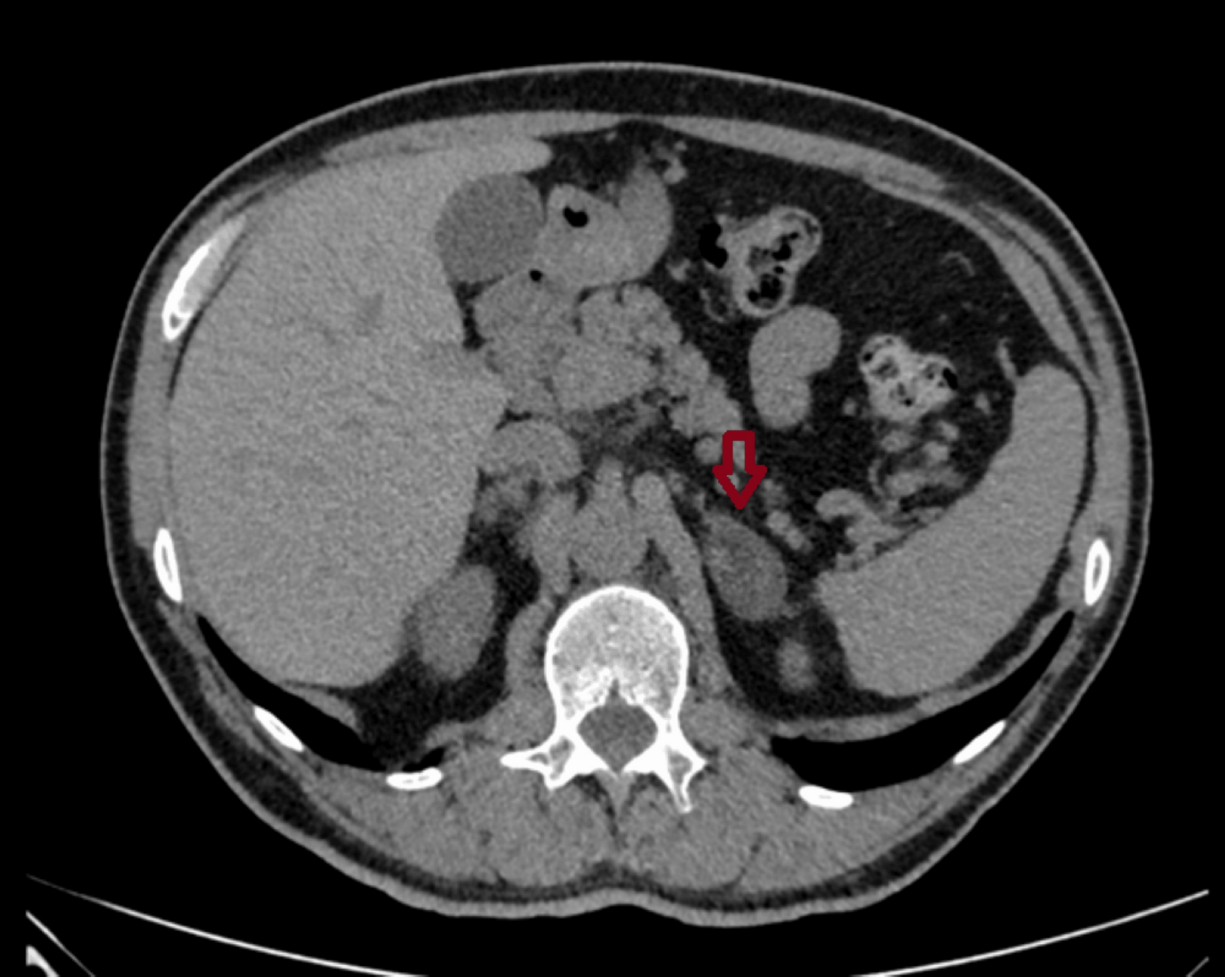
Adrenal imaging findings of Patient 3 An abdominal CT (transverse plane) of a 60-year-old man with bilateral adrenal incidentalomas (an incidentaloma in the left adrenal gland measuring 37×22 mm).
